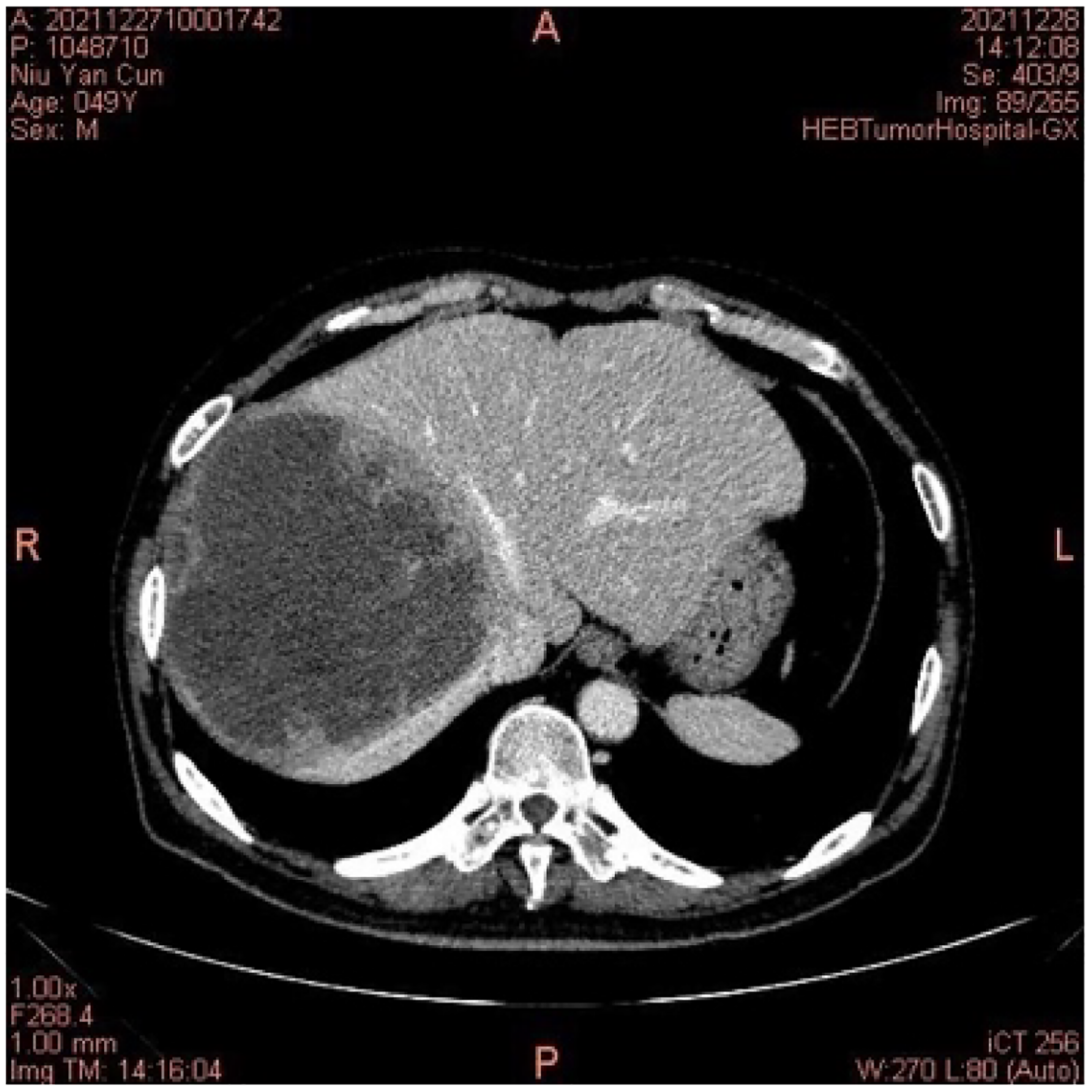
On 28 December 2021, the computed tomography of the abdomen indicated right lobe liver cancer.
